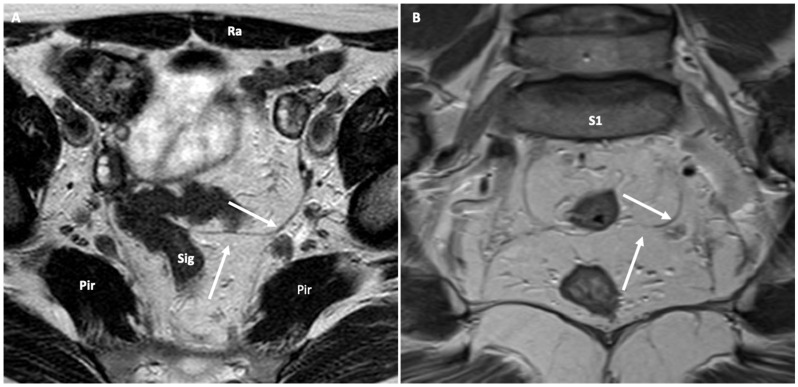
MR appearance of the H-shaped main linear condensation of endopelvic fascia (arrows) as seen on axial (A) and coronal (B) T2-weighted images to determine anatomical compartments and sub-compartments. Pir: piriformis muscle; Sig: sigmoid colon; Ra: rectum abdominis muscle; S1: 1st sacral bone.
